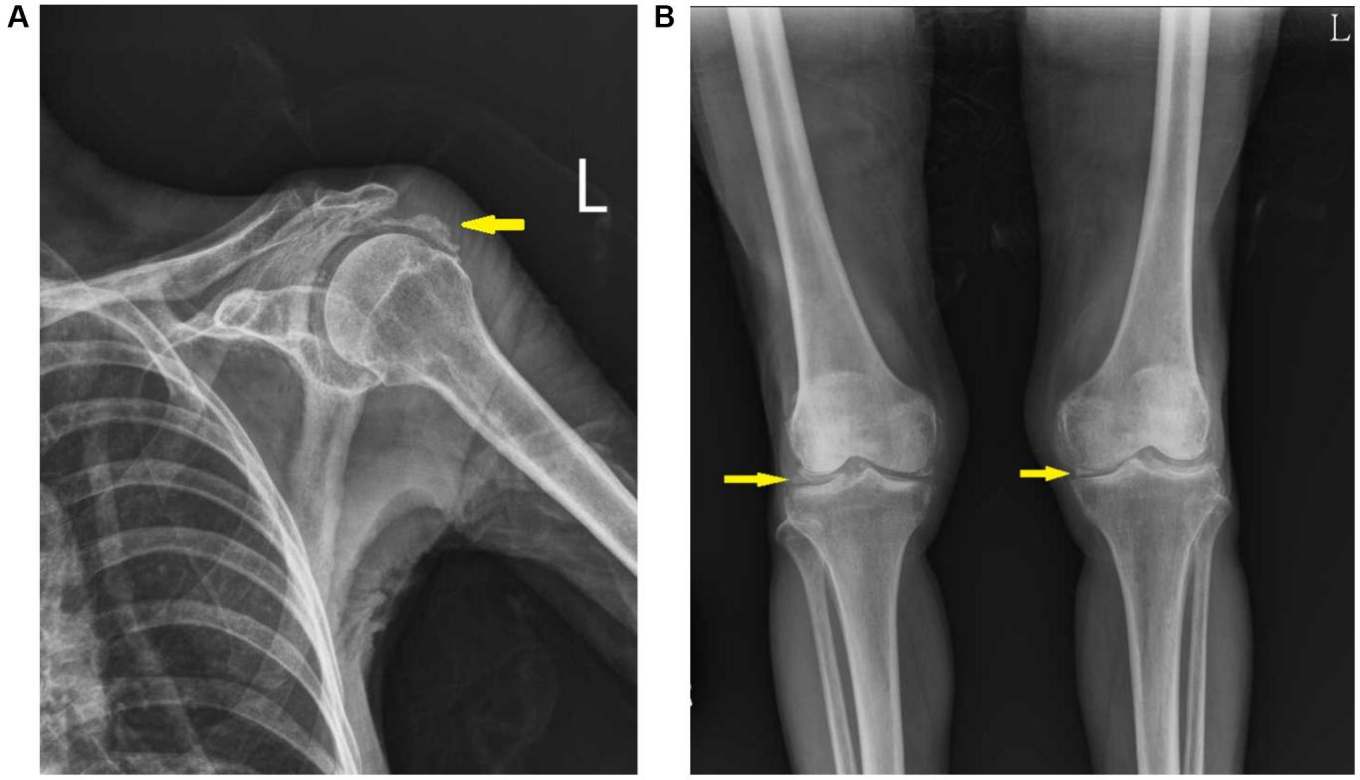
Radiographic image of the shoulder and knee joints. Calcifications were noted in the shoulder (A) and knee (B) joint space, compatible with chondrocalcinosis (yellow arrow).
